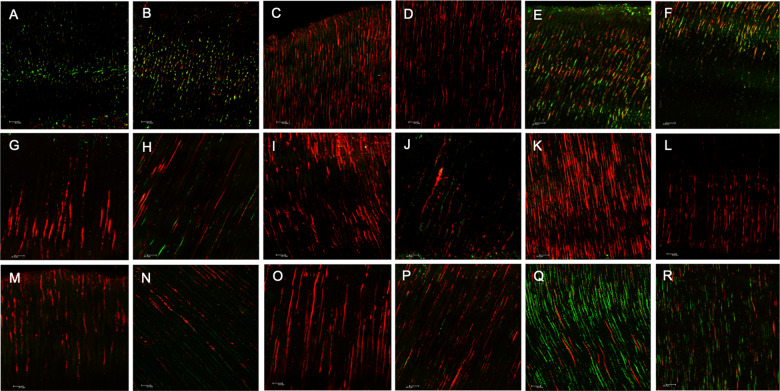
Images of contaminated dentin after using the medications at the superficial (left column) and deep areas (right column) (A and B). Calcium hydroxide with the vehicle propylene glycol, 7 days (G1). C and D Calcium hydroxide with the vehicle propylene glycol, 7 days, with ultrasonic agitation (G2). E and F Calcium hydroxide with the vehicle distilled water, 7 days (G3). G and H Calcium hydroxide with the vehicle distilled water, 7 days with ultrasonic agitation (G4). I and J Calcium hydroxide with the vehicle propylene glycol, 15 days (G5). K and L Calcium hydroxide with the vehicle propylene glycol, 15 days, with ultrasonic agitation (G6). M and N Calcium hydroxide with the vehicle distilled water, 15 days (G7). O and P Calcium hydroxide with the vehicle distilled water, 15 days, with ultrasonic agitation (G8). Q and R Positive control of the bacterial contamination without medication. These images are from the cervical third
